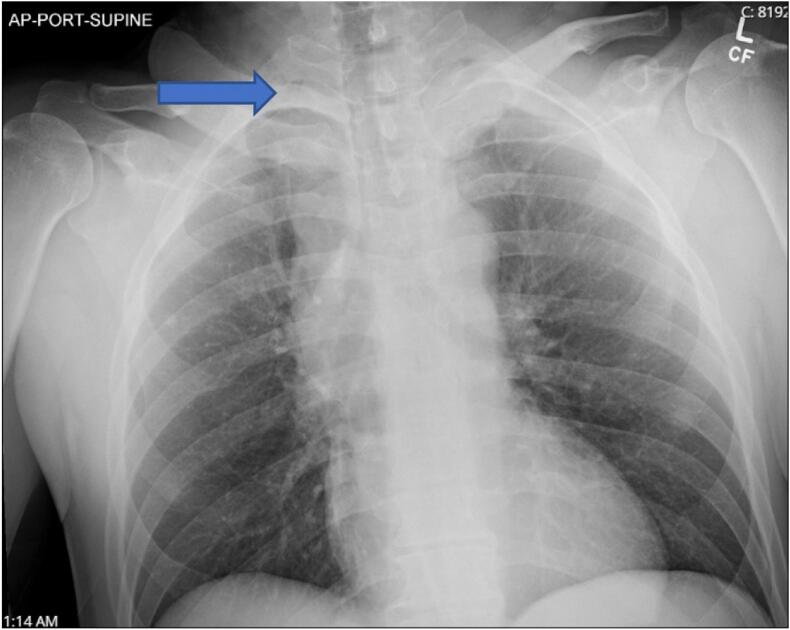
Chest X-ray on arrival to trauma center with right, apical pneumothorax.
